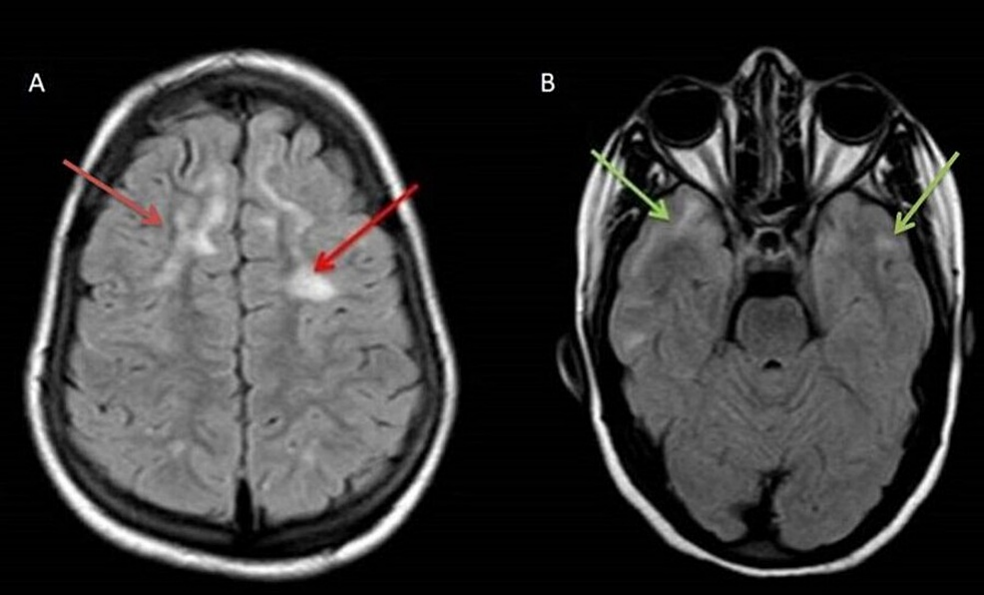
Axial fluid-attenuated inversion recovery (FLAIR) images (A) Confluent hyperintensities involving the white matter in B/L frontal lobes (red arrows). (B) Similar confluent hyperintensities involving white matter in B/L temporal lobes (green arrows). B/L: bilateral.
